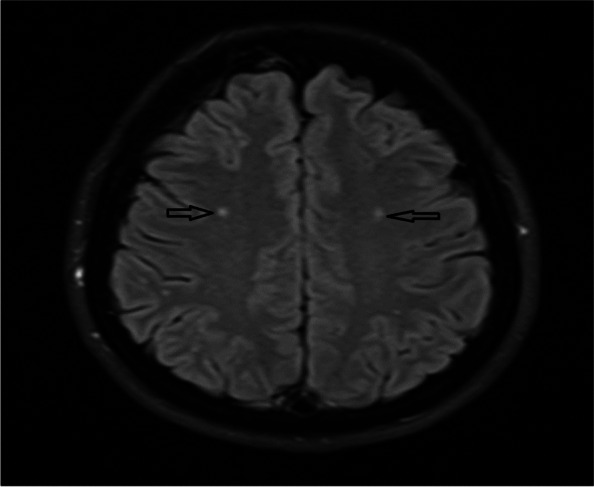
The white matter lesions in the cranial axial T2 (FLAIR) MRI in a patient with migraine (Black arrows showing the lesions)
